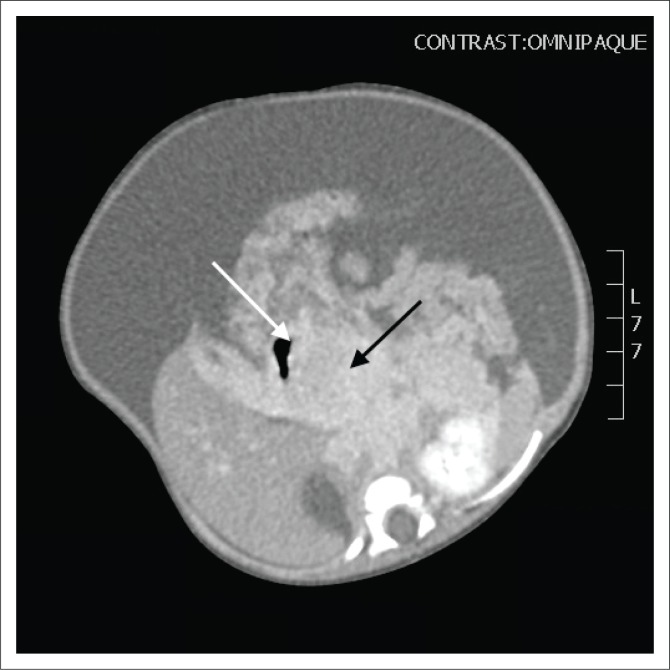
Axial post-contrast computed tomography image demonstrating the locally invasive pancreatic mass (black arrow) with a poor plane of separation from the D2 segment of the duodenum (white arrow).
